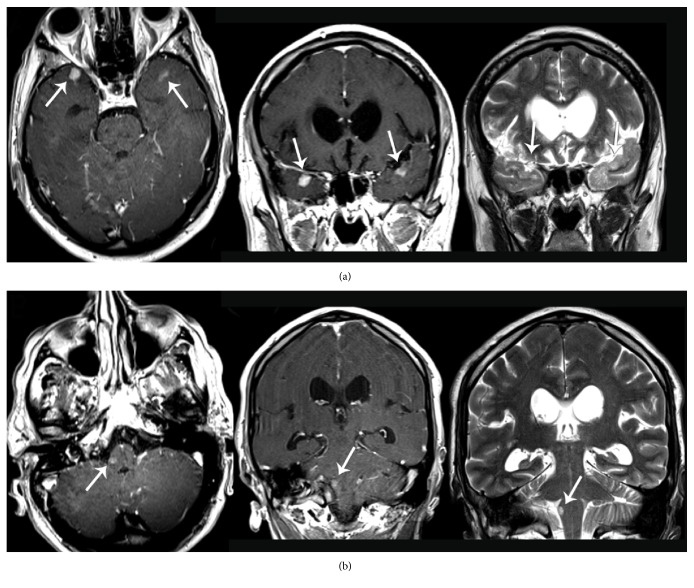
Contrast-enhanced T1- and T2-weighted brain MRI sequences showing contrast enhancing infracentimetric nodules of both temporopolar regions (line (a)) and of the brainstem (line (b)) (white arrow).
